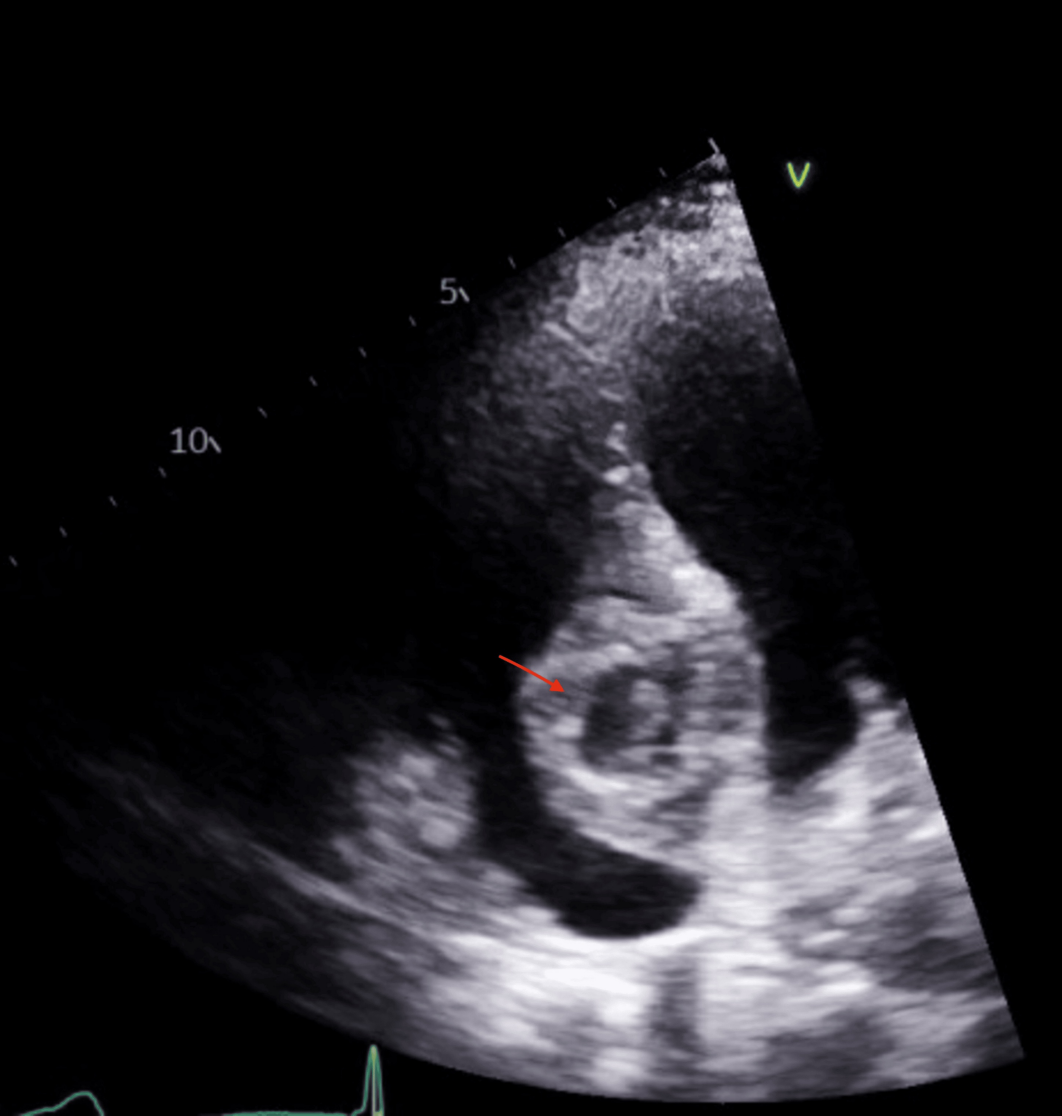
Apical four-chamber view on TTE showing complex cystic mass in the basal interventricular septum, close to the tricuspid annulus/right atrium TTE: transthoracic echocardiography
